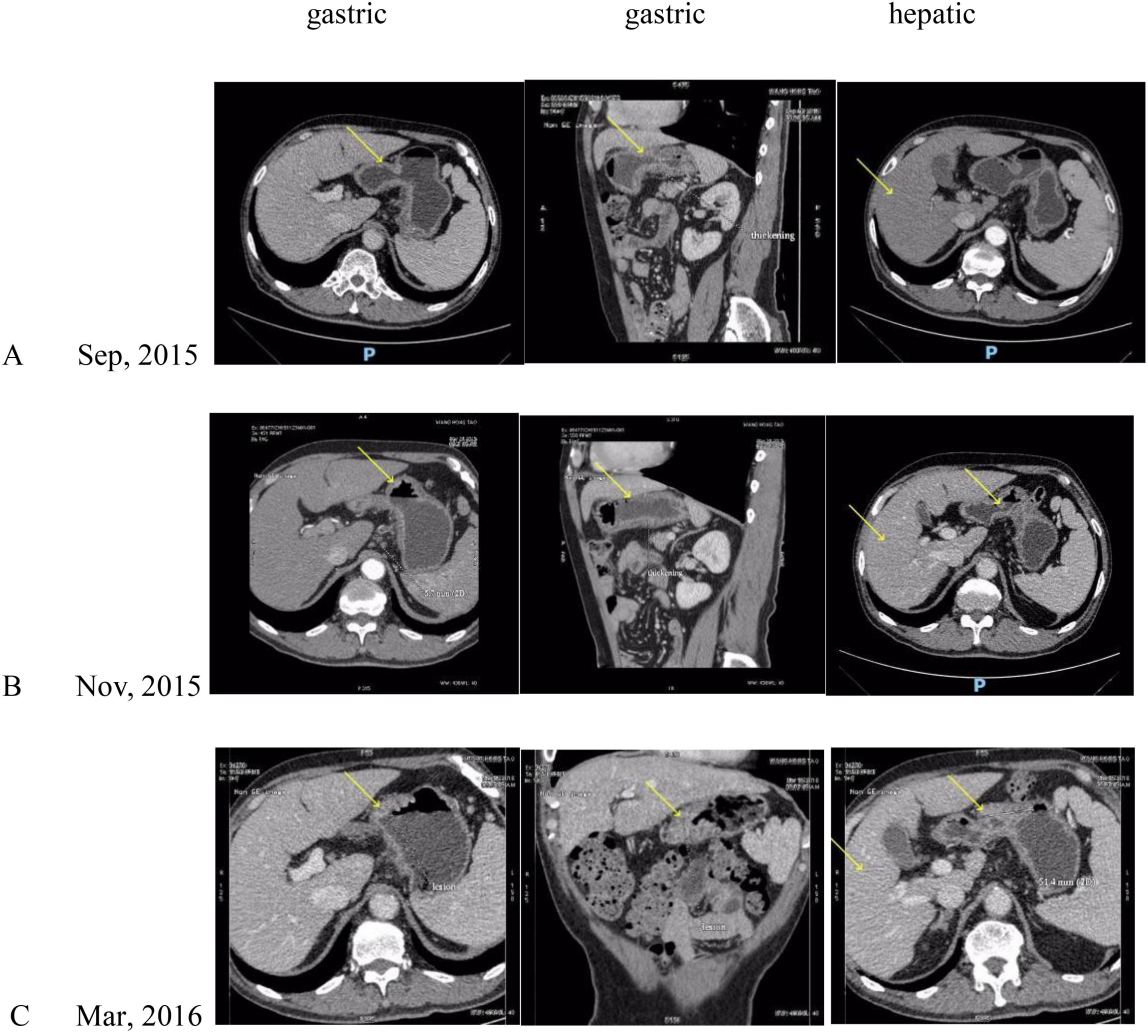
(A): The stomach and liver were scanned by CT at the first follow-up after S-1 monotherapy. (B): The stomach and liver were scanned by CT at the second follow-up after S-1 monotherapy. (C): CT of lesion progression. Yellow arrows indicate the location of the lesion.
